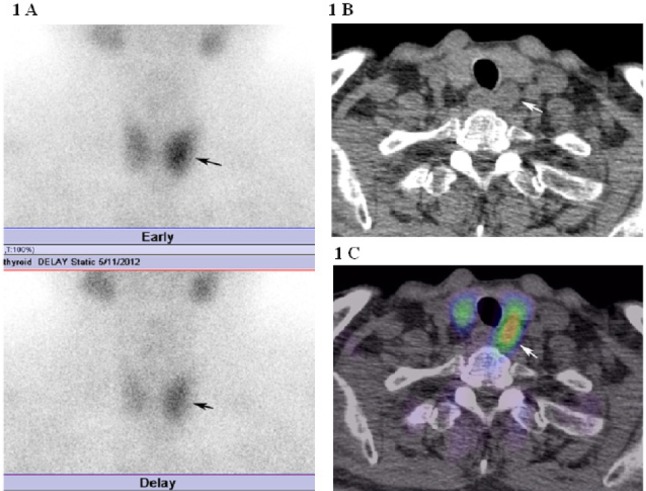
A. Initial and delayed phases of planar ^99m^Tc-MIBI scintigraphy showed increased activity in the left lobe (arrow). MIBI could not be used to differentiate between a parathyroid lesion and a thyroid lesion. B. Axial non-contrast enhanced CT of the neck. A lesion was detected posterior to the left lobe of the thyroid (arrow). C. Fusion axial image of SPECT/CT. The radiotracer accumulated in the lesion posterior to the left lobe of the thyroid (arrow). Adenoma of the left lower parathyroid gland was removed during surgery
